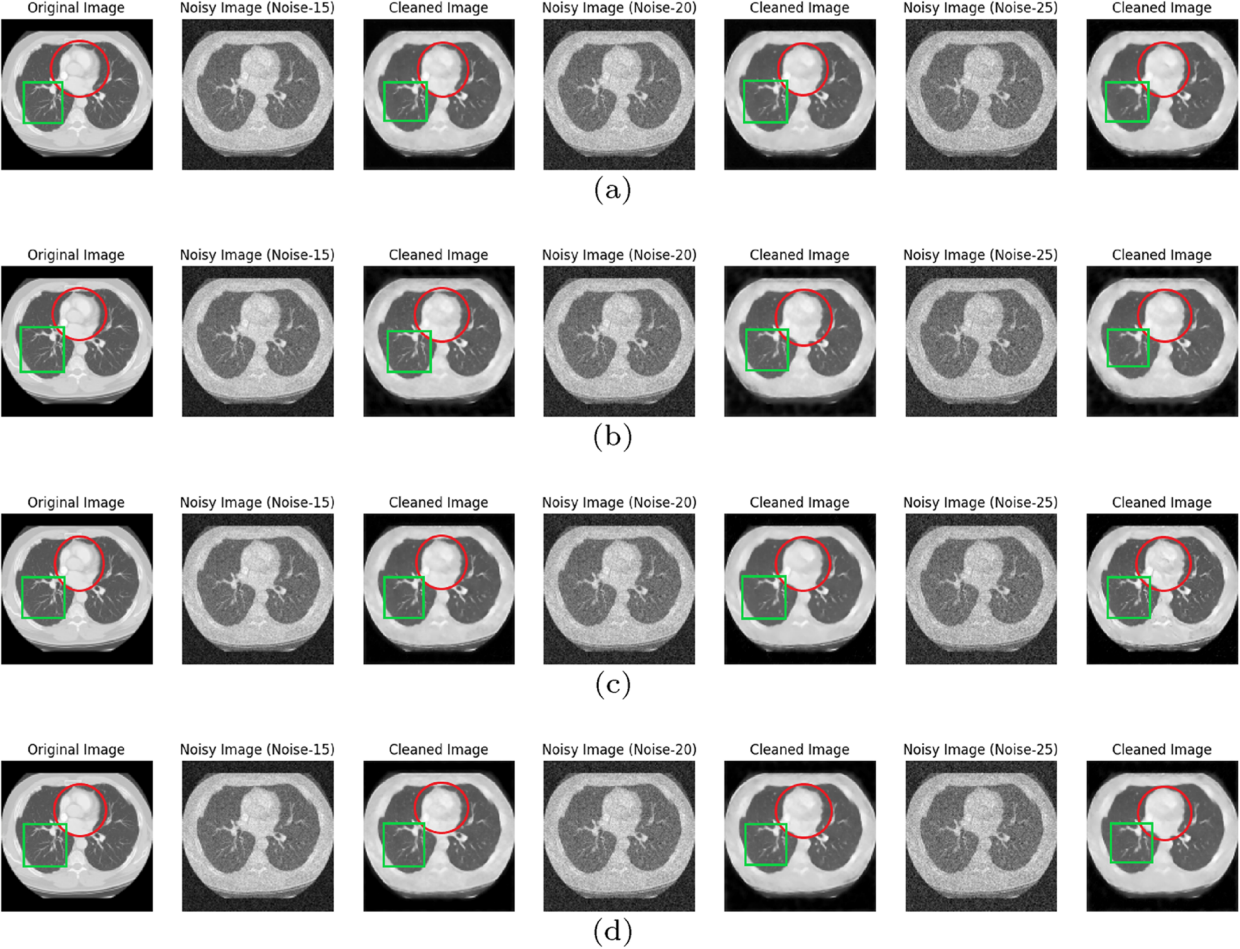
Original CT image with noisy($$N_{sp}$$=15,20,25 and $$N_{bl}$$=0 to 55) and denoised or cleaned image by DMF-Net where (a and c) shows for specific noise without and with augmentation in (b and d) shows for blind noise without and with augmentation; red circle and green box to observe inter lungs area and left lungs respectively with original image
